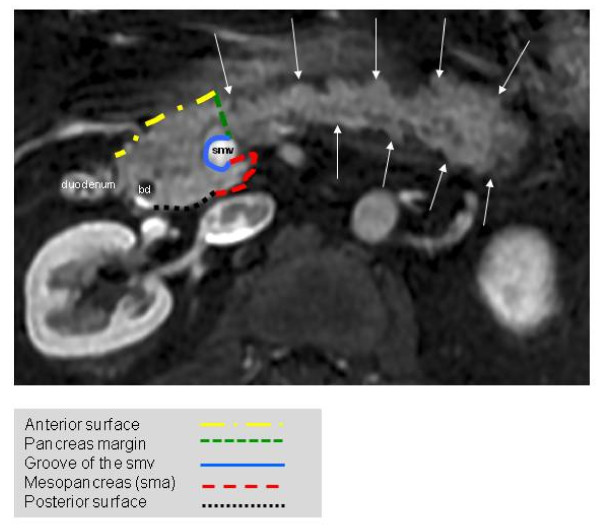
Magnetic resonance imaging of the pancreas indicating the circumferential resection margins (bd: bile duct, smv: superior mesenteric vein, arrows indicate pancreatic tail).
